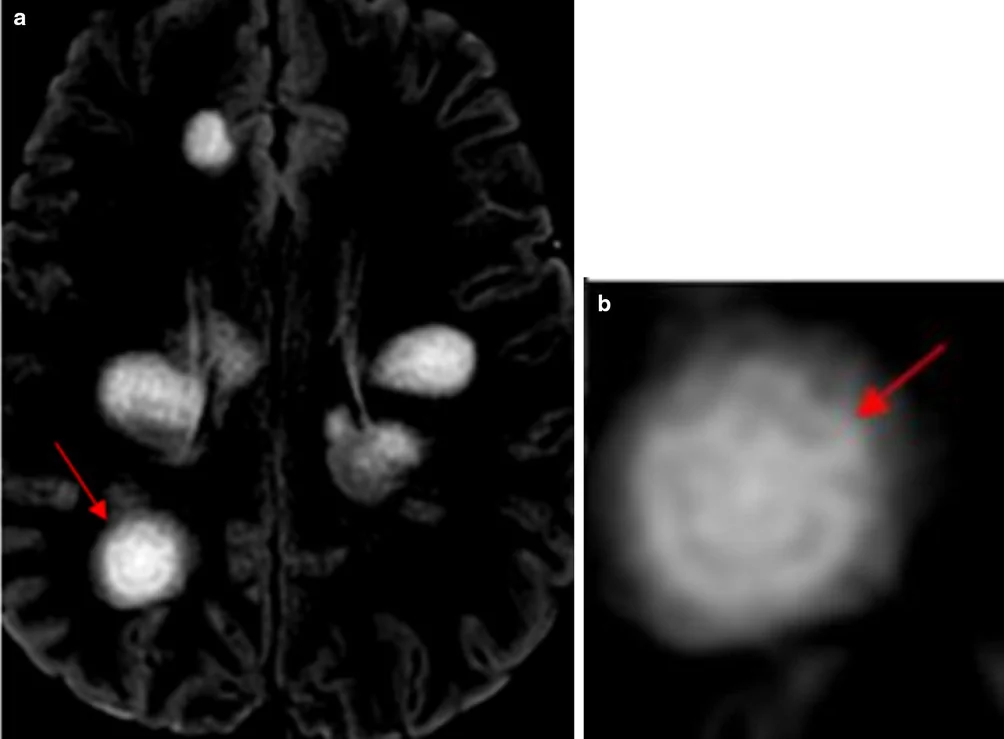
MRI Brain axial FLAIR view obtained on day 18 after symptom onset, showing multiple white matter lesions, with arrows denoting concentric layers of demyelination (a). Concentric layers are better seen with the magnified image (b).
